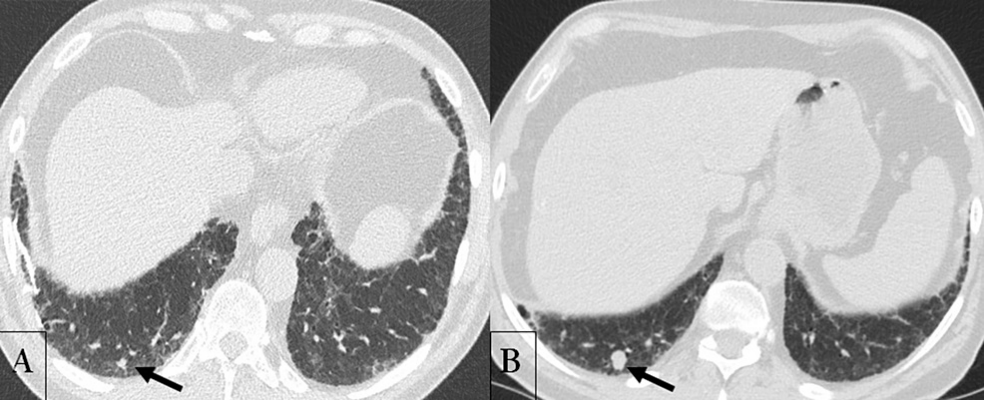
Unenhanced CT scan of the chest performed at one-year interval (A: June 2019; B: July 2020), showing the increase in size of a solitary lung nodule (arrow) located within the posterobasal segment of the right lung, from 4 mm to 15 mm.
